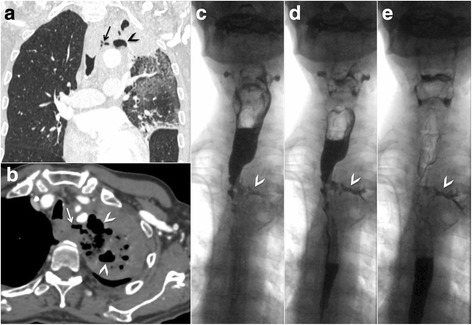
a, b Coronal and axial thoracic contrast-enhanced computed tomographic scans (lung and soft tissue window, respectively) show a condensation of the apical segment of the left upper lobe with parenchymal excavations (*arrowheads*) and extended pulmonary infiltrations involving the whole lobe. A fistula between the esophagus and left upper lung is suspected due to the presence of air in the mediastinum (*arrows*). c–e Videofluoroscopic studies showing contrast leakage through a fistulous tract (*arrowheads*) originating from the upper esophagus and progressively joining the left apical lung without contrast bronchogram, thus confirming the esophagopulmonary type of fistula
